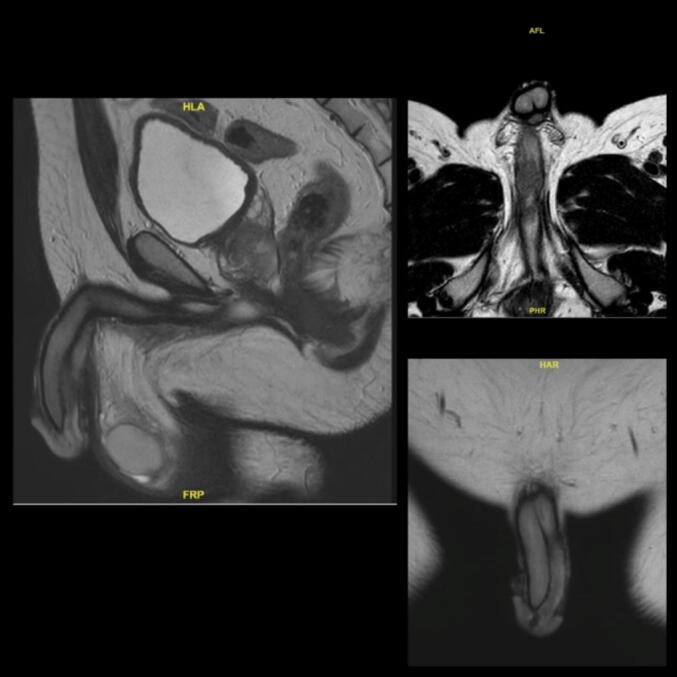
T2- weighted sequence of MRI Pelvis depicting the disease in bulbar urethra at baseline.
